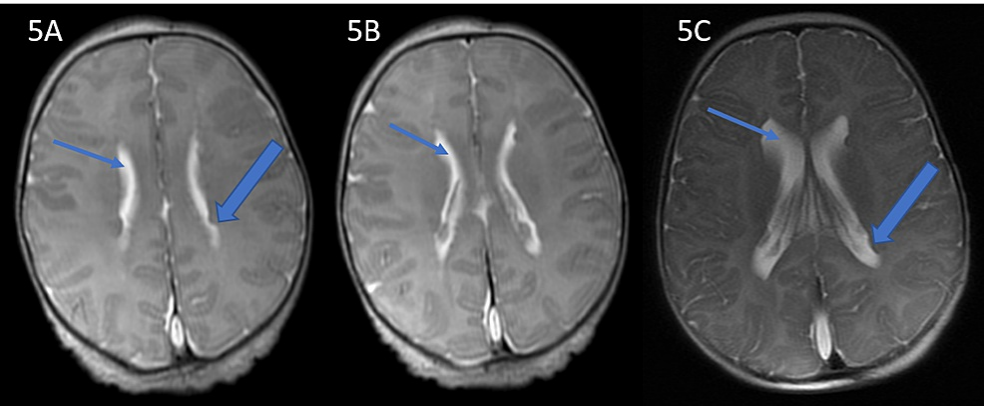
Ventricular size increase and heterotopia T2-weighted axial MRI of the brain on day 5 of life (A, B) and at 15 weeks of life (C) show interval mild increase in size of lateral ventricles (thin arrow) in addition to subependymal heterotopia (thick arrow).
